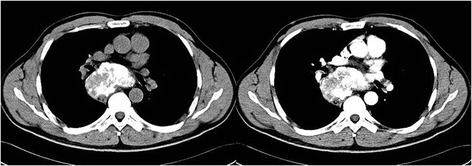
Chest unenhanced CT scan shows a heterogeneous mass with calcification located in the posterior mediastinum. Its border was clearly demarcated, and enhanced CT scan shows the mass with little enhancement.
